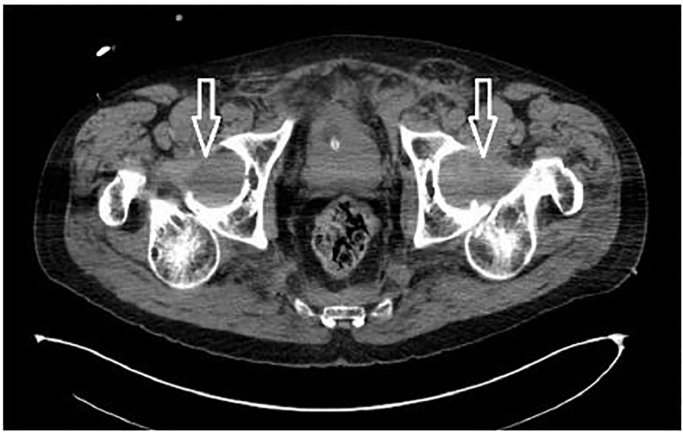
Computed tomography axial image demonstrating bilateral posterior hip dislocations (arrows).
